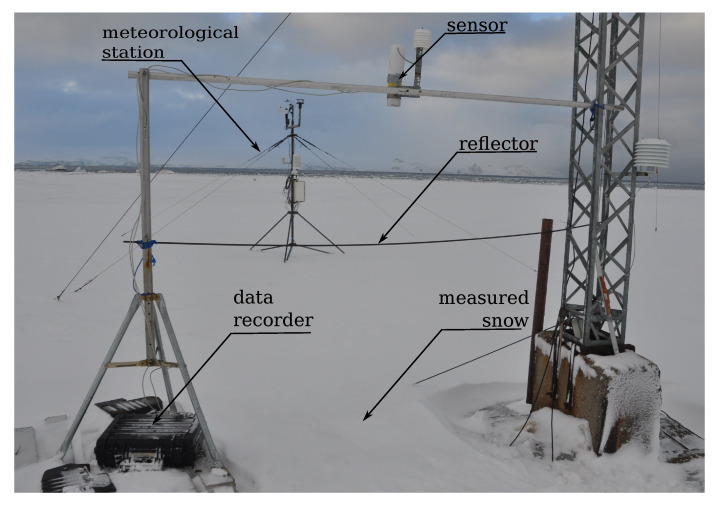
Measurement setup. In first plane: ultrasonic sensor with reflector installed. In second plane: Automatic Weather Station.
